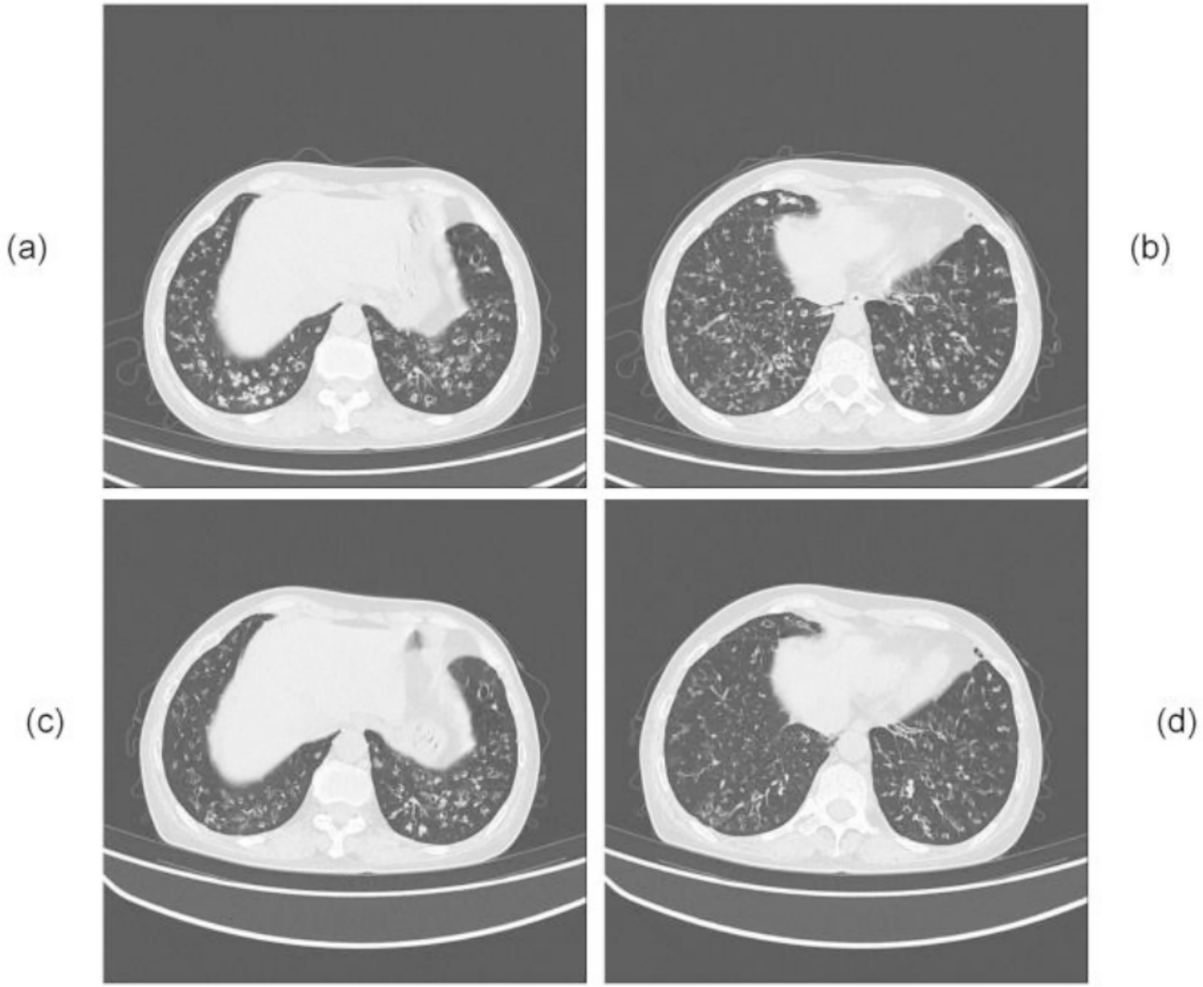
Chest CT of Case 2. Prior to treatment initiation: (a,b) multiple nodular opacities are seen in both lung fields, more prominent in the right lung, accompanied by distorted lung markings and extensive, heterogeneous patchy consolidations and ground-glass opacities in the subpleural regions. One month after treatment. (c,d) The number and extent of bilateral nodular opacities have significantly decreased. Marked improvement is noted in the distorted lung markings of the right subpleural area, demonstrating overall definite improvement compared to the pretreatment scan.
